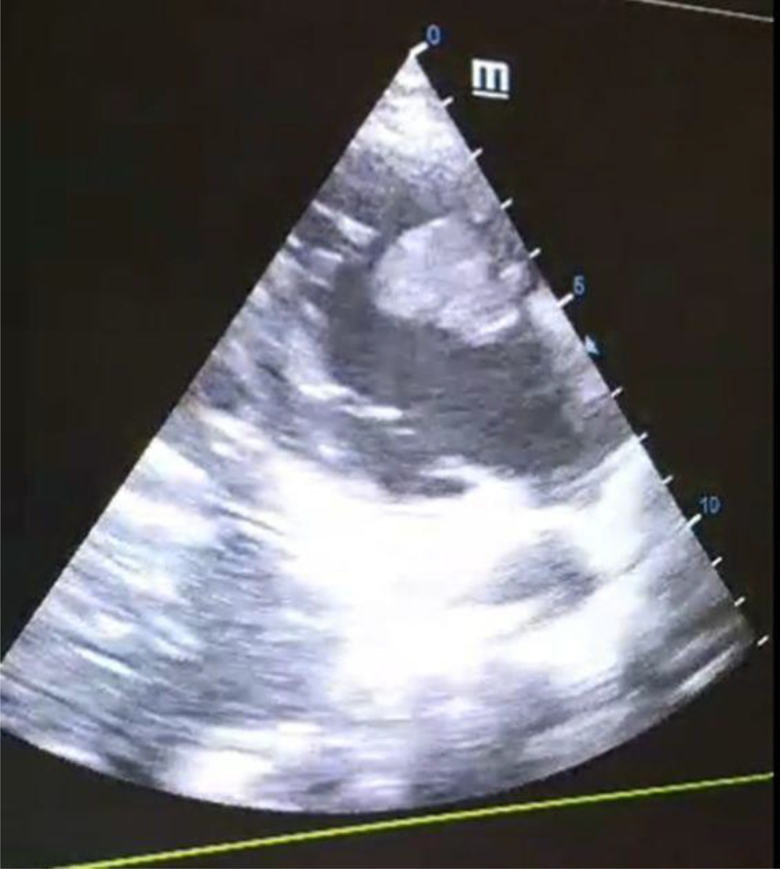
Transthoracic echocardiogram. The image shows a large, well-defined mass (intracardiac thrombus) within the right ventricle.
